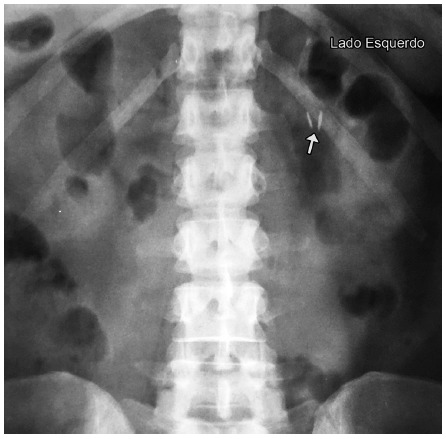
Simple abdominal radiographic study revealing the two clips in expected normal position: to the left of the spine
